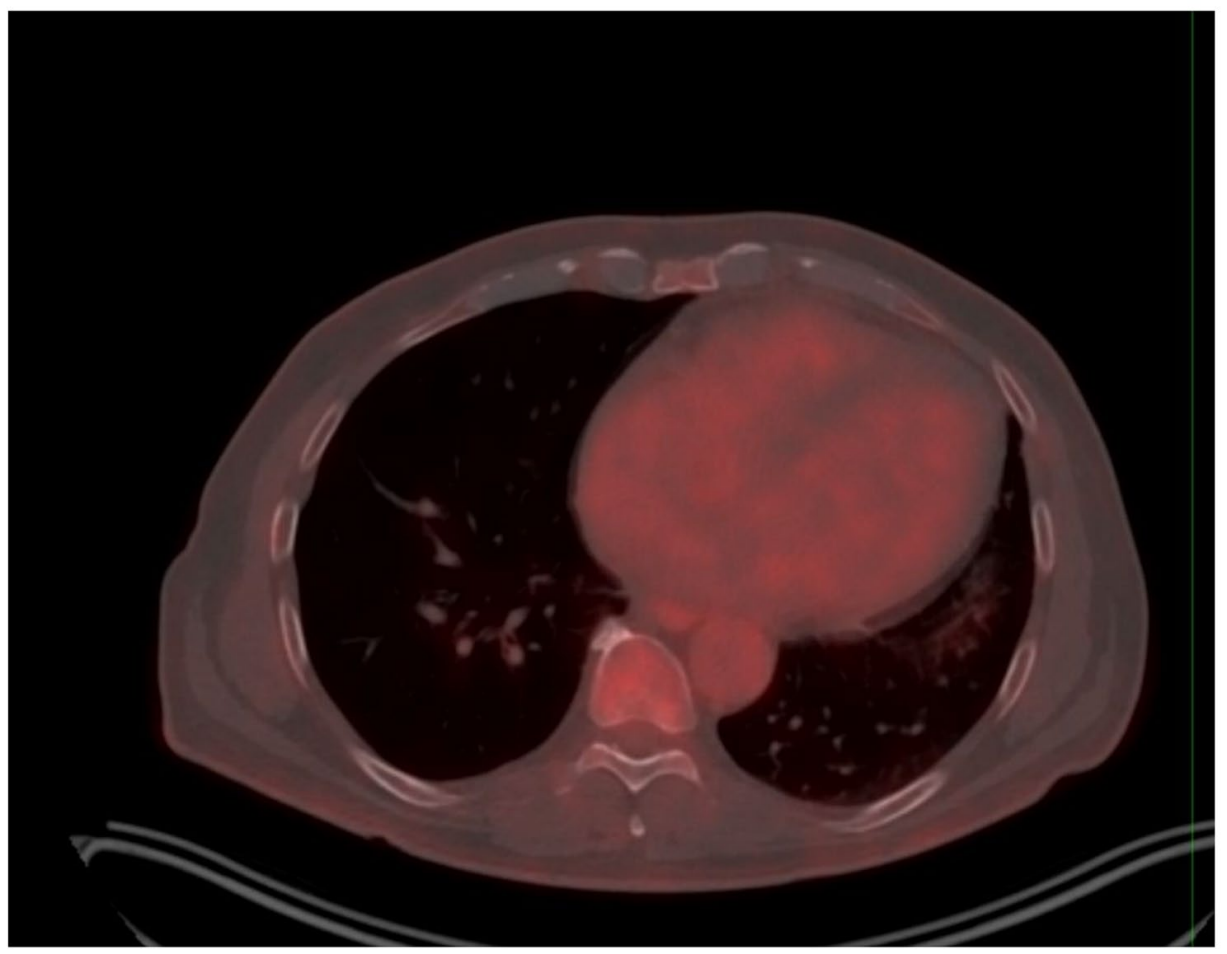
Figure 4. Legend: PET CT imaging. No abnormal FDG uptake in the left ventricular myocardium to suggest active inflammation
